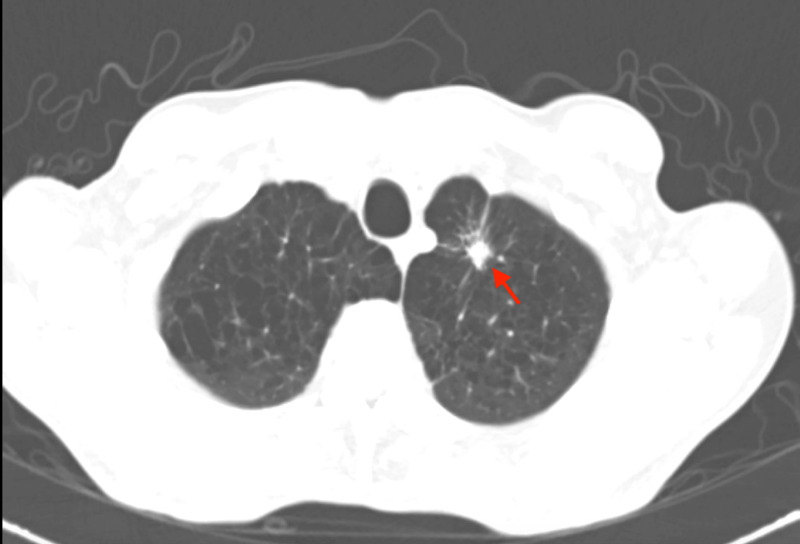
CT chest showing left upper lobe lesion (arrow) CT: computed tomography
